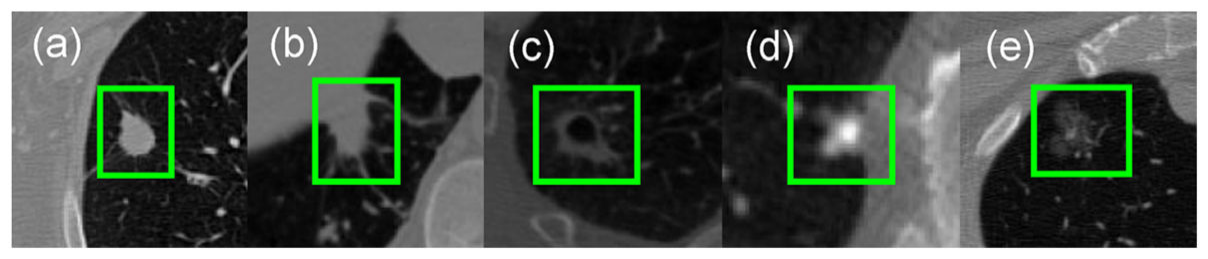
Example images of lung nodules with different locations and shapes in CT: (a) common isolated nodule. (b) juxtapleural nodule. (c) cavitary nodule. (d) calcific nodule. (e) ground-glass opacity (GGO) nodule.
